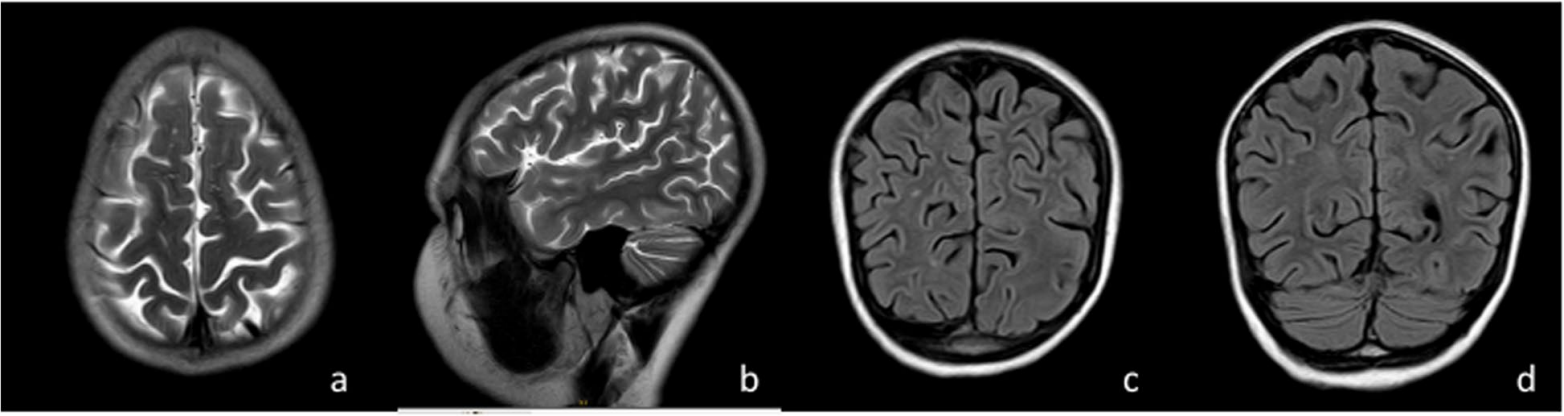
Head MRI (a T2WI axial image, b T2WI sagittal image, c T2-FLAIR axial image, d T2-FLAIR coronal image) showed multiple spot-like and nodular abnormal signals in both cerebral hemispheres, slightly widened bilateral lateral ventricles, and slightly deepened sulci in both cerebral and cerebellar hemispheres
